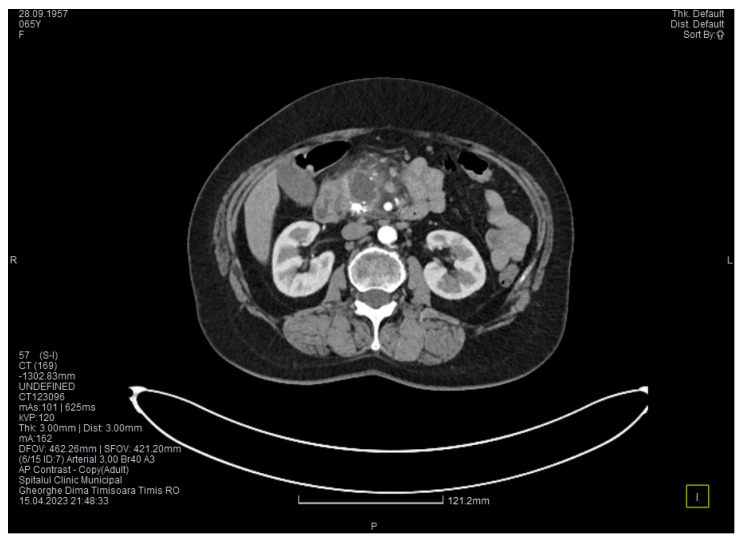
Initial CT scan shows a cyst in the pancreatic head.
